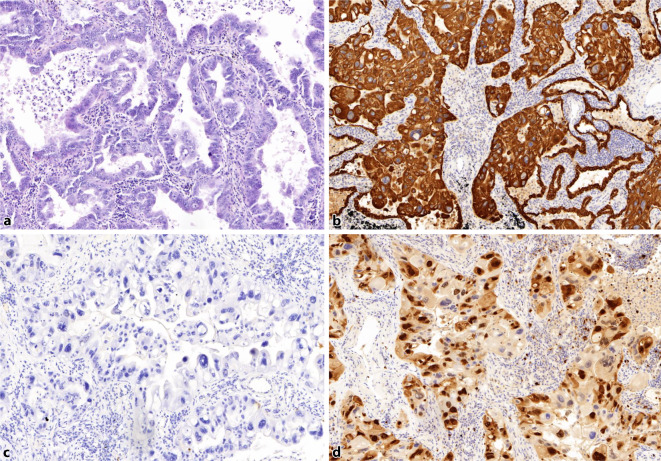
Nach Resektion des Tumors bestanden die symptomatischen Hypoglykämien zunächst weiter, sodass ein massenhafter Glukoseverbrauch durch die Tumorlast selbst ausgeschlossen werden konnte und somit der Verdacht auf eine IGF-2-induzierte Hypoglykämie im Sinne eines paraneoplastischen Syndroms bestand. Da in diesem Falle ein peripher erhöhter Glukoseverbrauch der Muskulatur in Ruhe zu finden sein müsste, erfolgte postoperativ eine ^18^F‑FDG-PET-CT (Fluordesoxyglukose). Diese konnte eine pathologisch gesteigerte Aufnahme der markierten Glukose in die quergestreifte Muskulatur nachweisen (Abb. 6). Es wurde eine Chemotherapie mit Carboplatin, Pemetrexed und Pembrolizumab eingeleitet, worunter die Hypoglykämien sistierten.
